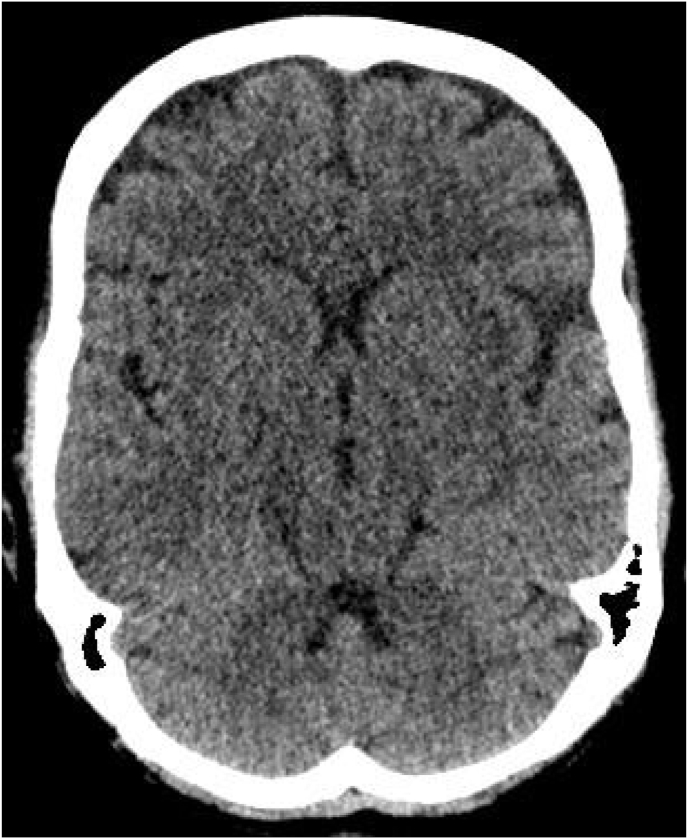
Normal axial non-contrast CT of brain.
